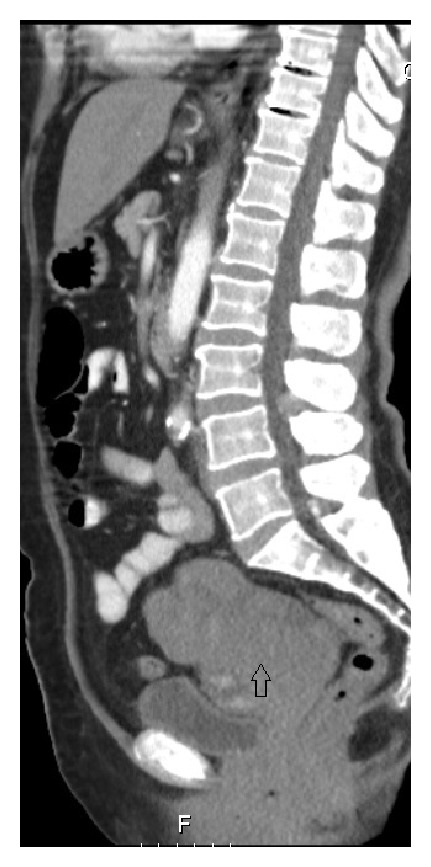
A CT scan showing a large mass of the left ovary.
